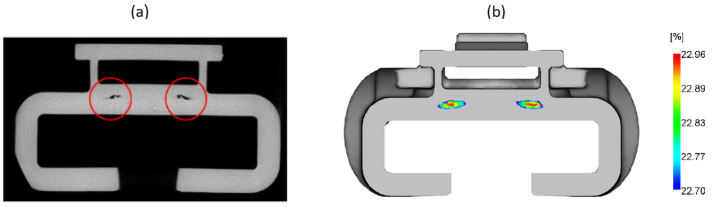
Location of the voids: (a) 3D X-ray slice of FH part, (b) Prediction of volumetric shrinkage at the void locations.
